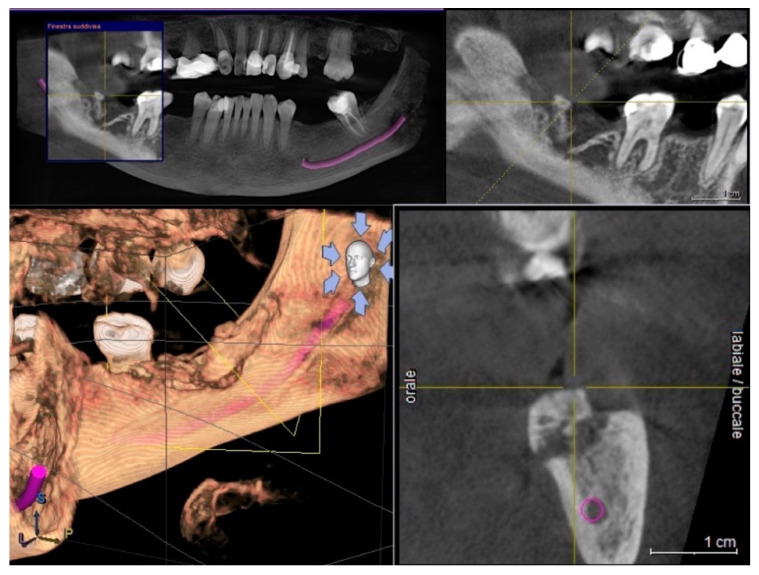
Cone beam-computed tomography showing the necrotic bone extension in a panoramic view with and without zoom, 3D rendering and cross-sectional view.
